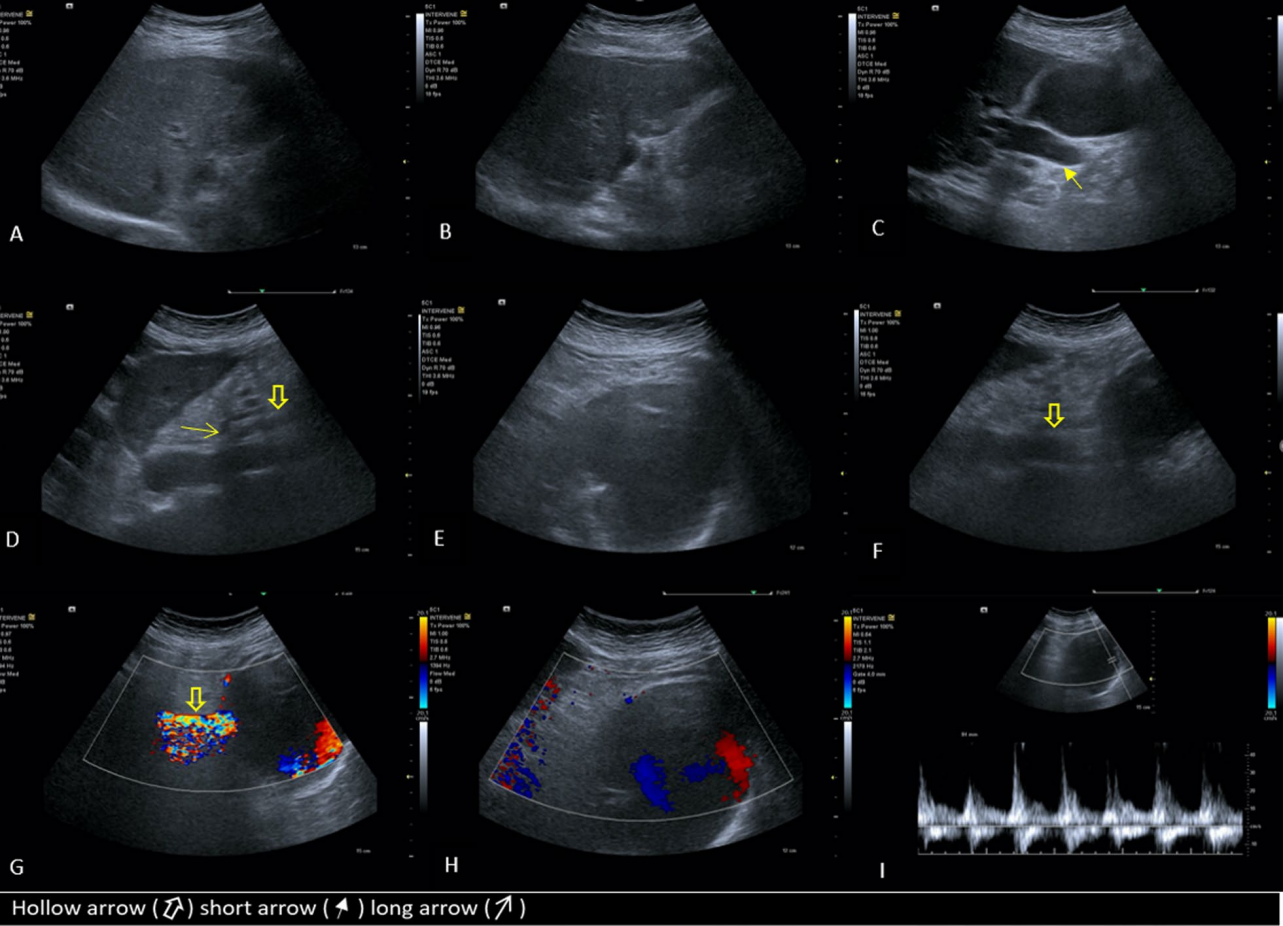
Surface of the liver is irregular and its echogenicity is mildly coarse and heterogeneous. Mild ascites is seen around the liver (A, B). Common bile duct and intrahepatic bile duct dilation (C). Sagittal scan of aorta shows stenosis of proximal of celiac trunk (D). The huge aneurysm with “yin and yang” sign on color Doppler ultrasound, and turbulent arterial blood flow on Spectral Doppler (E, H, I). The aneurysm is superiorly connected to superior mesenteric artery. The superior mesenteric artery continues its course on the anterior surface of the aneurysm to supply the small bowel (F, G). Hollow arrows shows superior mesenteric artery, short arrow shows dilated common bile duct, and long arrow shows celiac trunk
